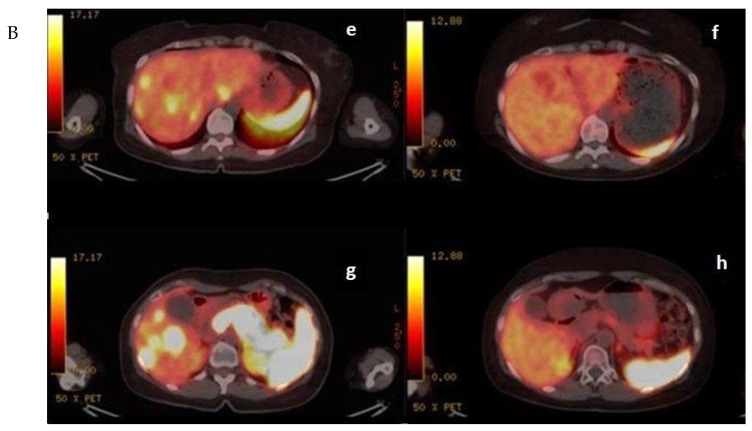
(A) (upper) Downstaging tumors (complete response) after metronomic TMZ–bevacizumab combination therapy. MRI images for the patient with metastatic NET before (a,c) and six months after treatment (b,d); (B) (bottom) downstaging tumors (complete response) after metronomic TMZ–bevacizumab combination therapy. Gallium-68 (Ga-68)-DOTATATE positron emission tomography (PET) images for the patient with metastatic NET before (e,g) and one year after treatment (f,h).
